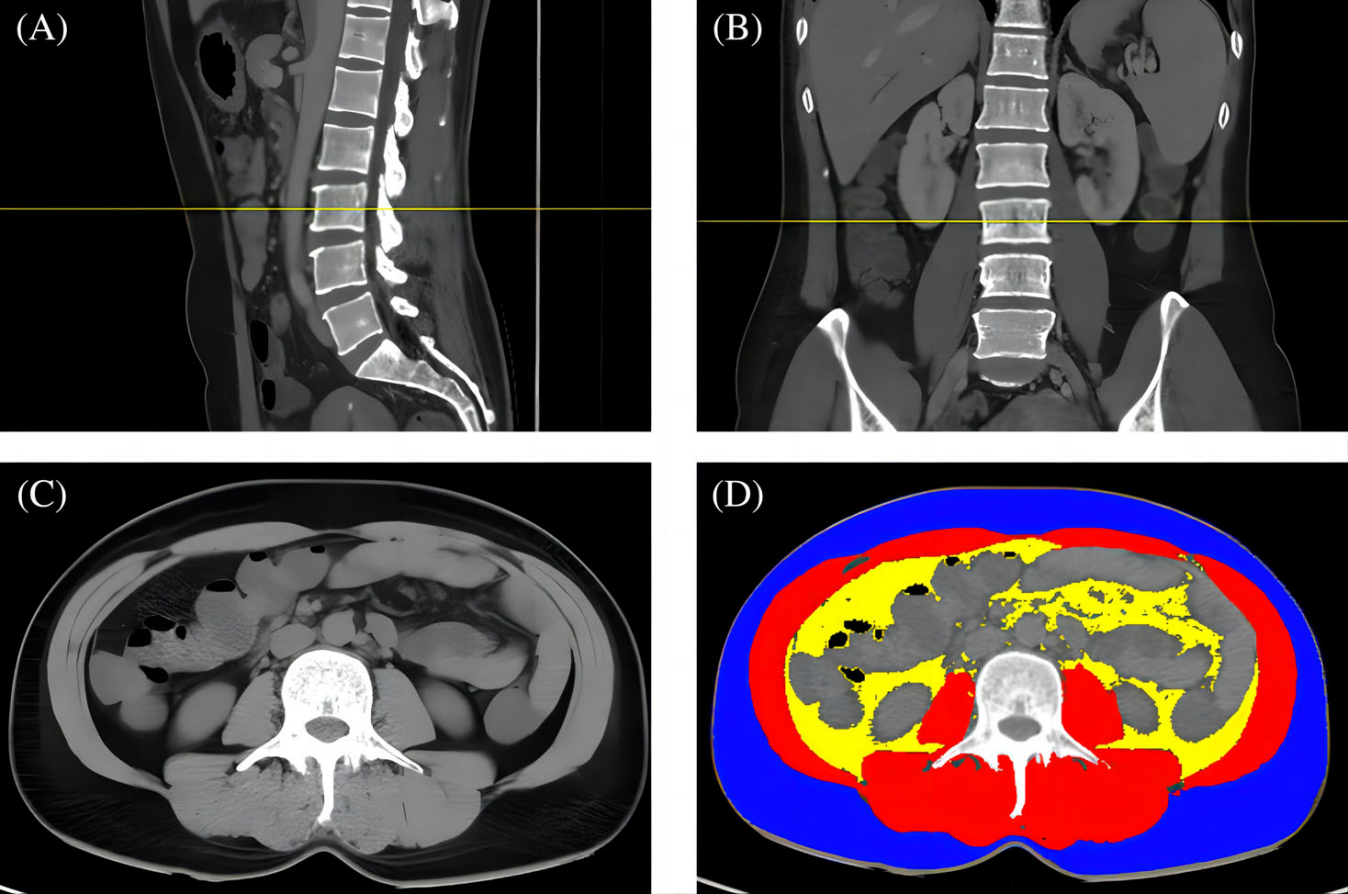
Computed tomography measurement of skeletal muscle area at the third lumbar vertebra (L3). (A) The middle segment of the third lumbar vertebra (L3) in the sagittal plane is marked with a yellow line; (B) The middle segment of L3 in the coronal plane is marked with a yellow line; (C) Horizontal cross section at L3; (D) TAMA was measured with a threshold from −29 to +150 HU (red); SAT was measured with a threshold from −190 to −30 HU (blue); and VAT was measured with a threshold from −150 to −50 HU (yellow). HU, Hounsfield unit; SAT, subcutaneous adipose tissue area; TAMA, total abdominal muscle area; VAT, visceral adipose tissue area.
